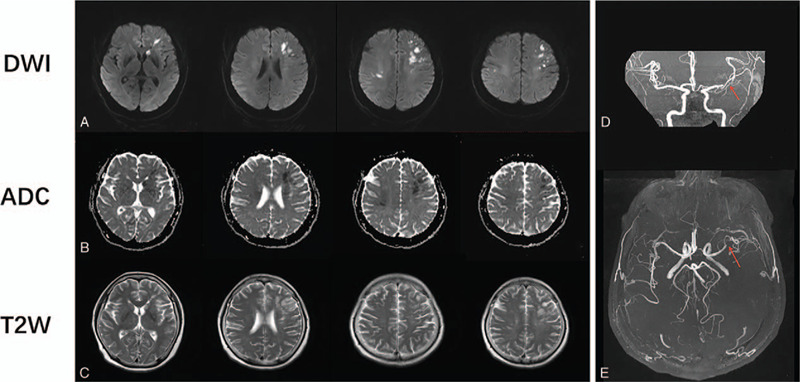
Repeat brain MRI (2 days after intravenous methylprednisolone treatment) results confirm an acute infarction in both frontal lobe hemispheres, the left basal ganglia, and the semioval center (bilateral). Diffusion weighted imaging results confirmed the presence of high-signal lesions (A); the T2-weighted results also revealed a slightly hyperintense signal (C). The corresponding lesions present as a hypointense signal on the apparent diffusion coefficient sequence. Magnetic resonance angiography revealed the existence of localized stenosis in the M1 segment of the left middle cerebral artery, as well as multiple intracranial arteriosclerosis (red arrow, D–E).
